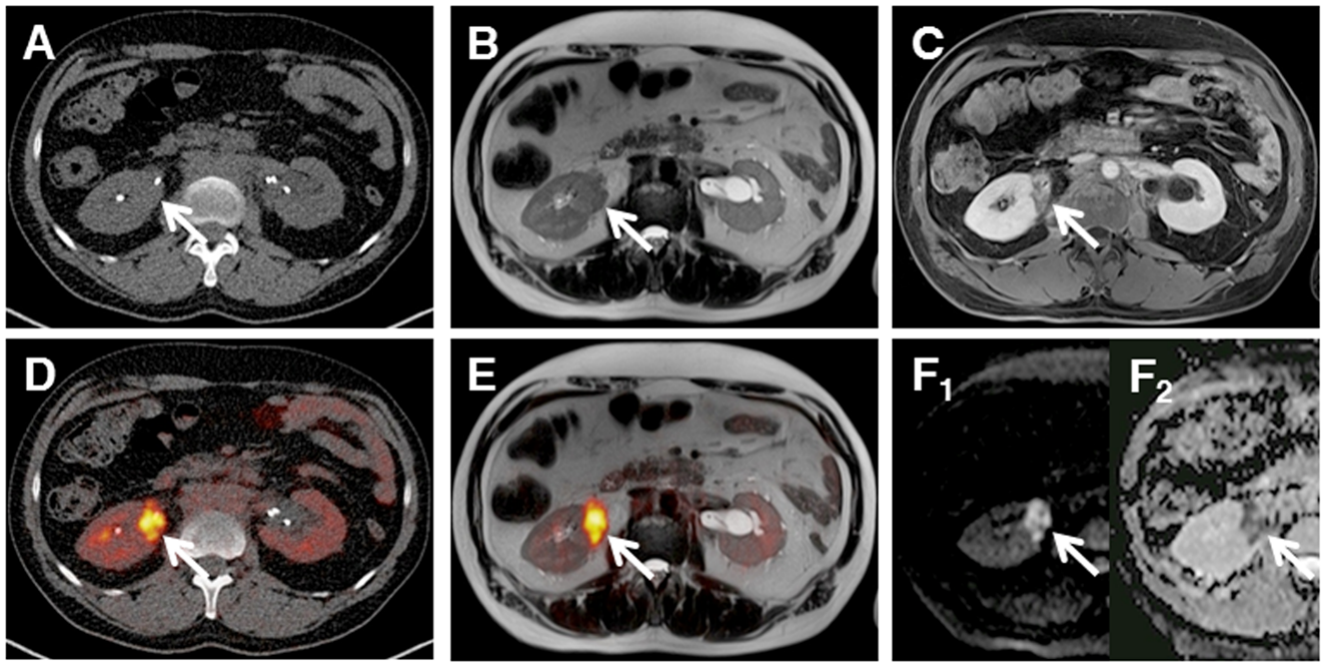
Manifestation of Lymphoma within the renal pelvis (arrows), which is not clearly visible on low-dose CT images (a), but can be identified on MR images (T2w Haste ax., b; T1w VIBE ax. post-contrast, c). The identical lesion reveals pathological glucose metabolism after image fusion on PET/CT (d) and PET/MRI (e) as well as high signal in DWI (f_1_) and a signal drop on the ADC-map (f_2_).
